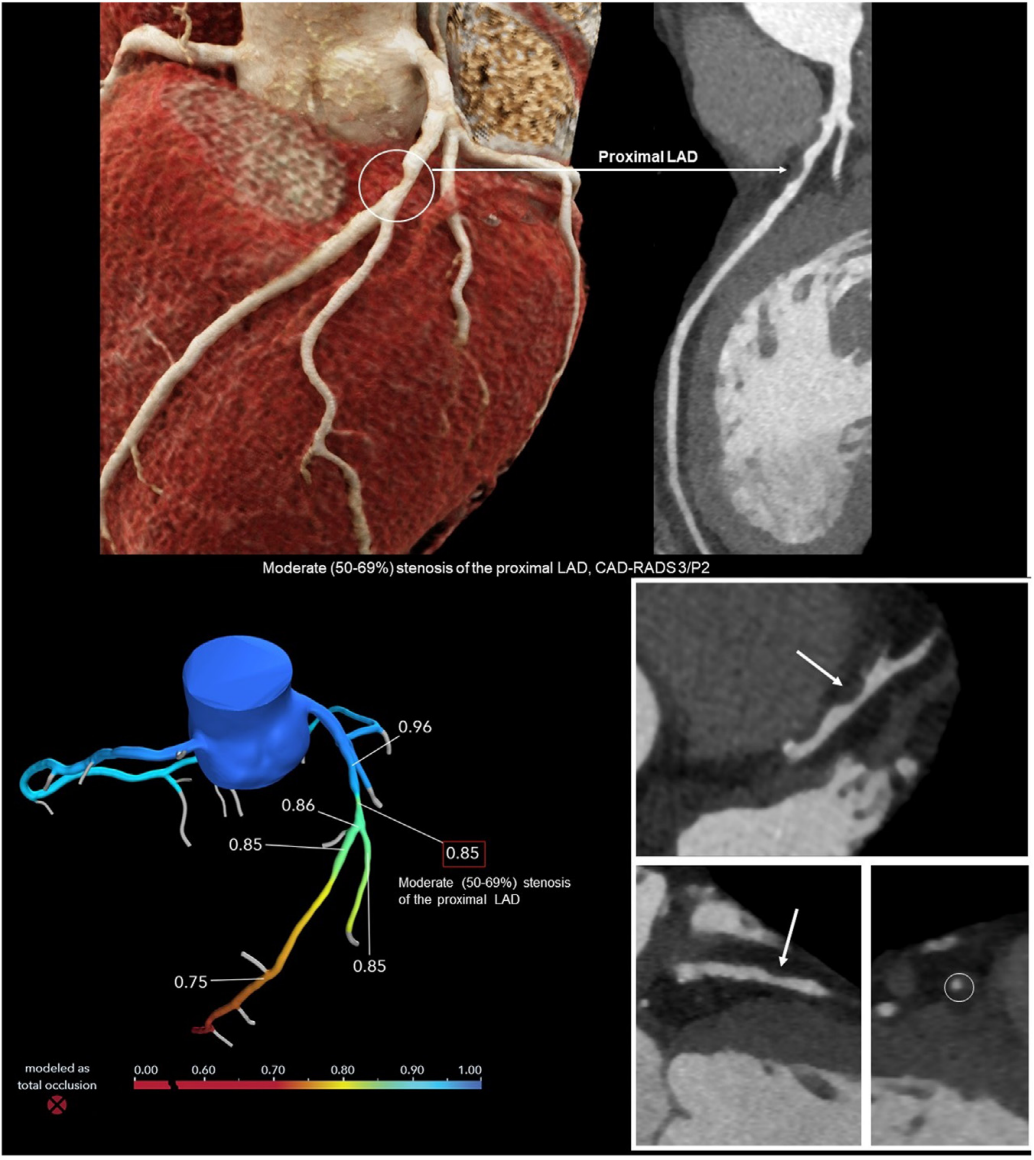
CT-derived fractional flow reserve (FFR_CT_) of the left anterior descending artery with moderate stenosis of the proximal LAD with a negative FFR_CT_.
